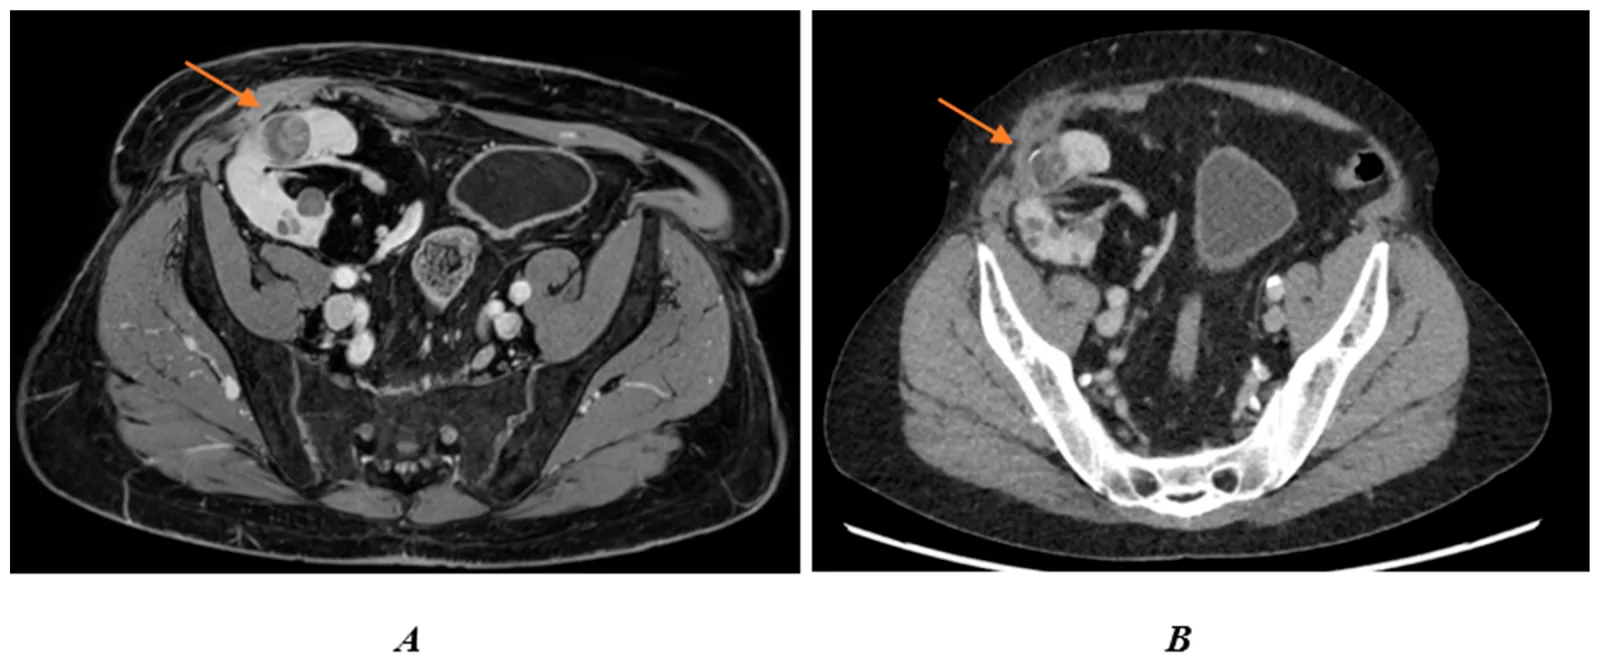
(A,B) Long-term radiological follow-up after CT-guided cryoablation. (A) MRI obtained 1 year after treatment showing post-ablation changes in the renal allograft lesion without residual disease. (B) Contrast-enhanced CT scan at 3-year follow-up confirming the absence of local recurrence.
